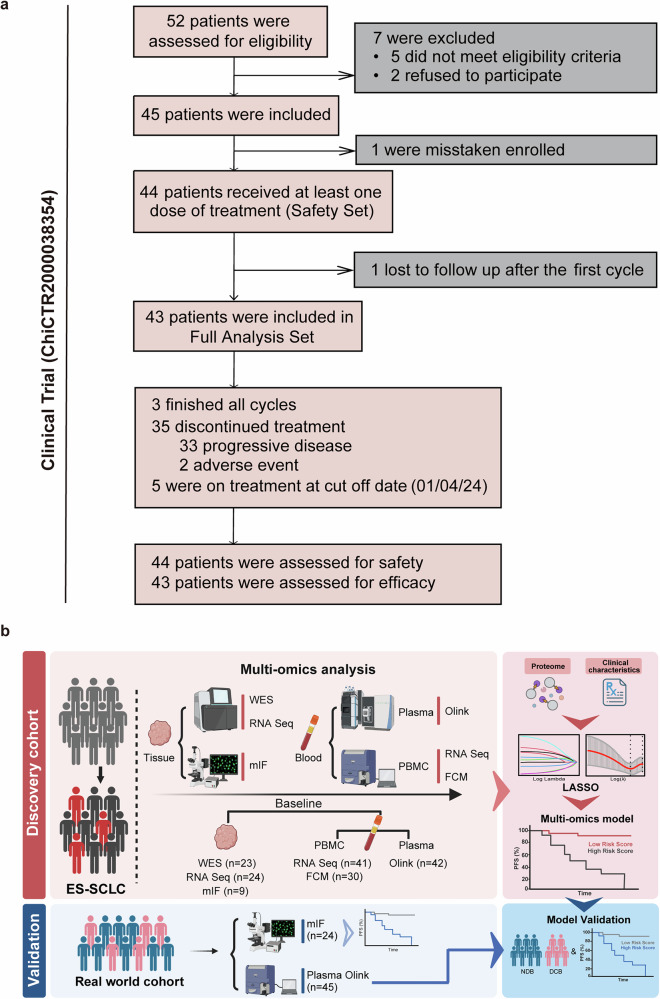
Study design. a. Of a total of 52 patients screened for study eligibility, five did not meet the inclusion criteria, two declined to participate and one was mistakenly enrolled. A total of 44 enrolled patients accepted at least one cycle of sintilimab plus chemotherapy and were assessed for safety, while 43 patients were evaluable for radiographic response assessment and efficacy. b. Design of the sample collection for biomarker research. Tumor samples and blood were collected at baseline. Validation of findings on the real-world cohort 1 (*n* = 24) and real-world cohort 2 (*n* = 45)
